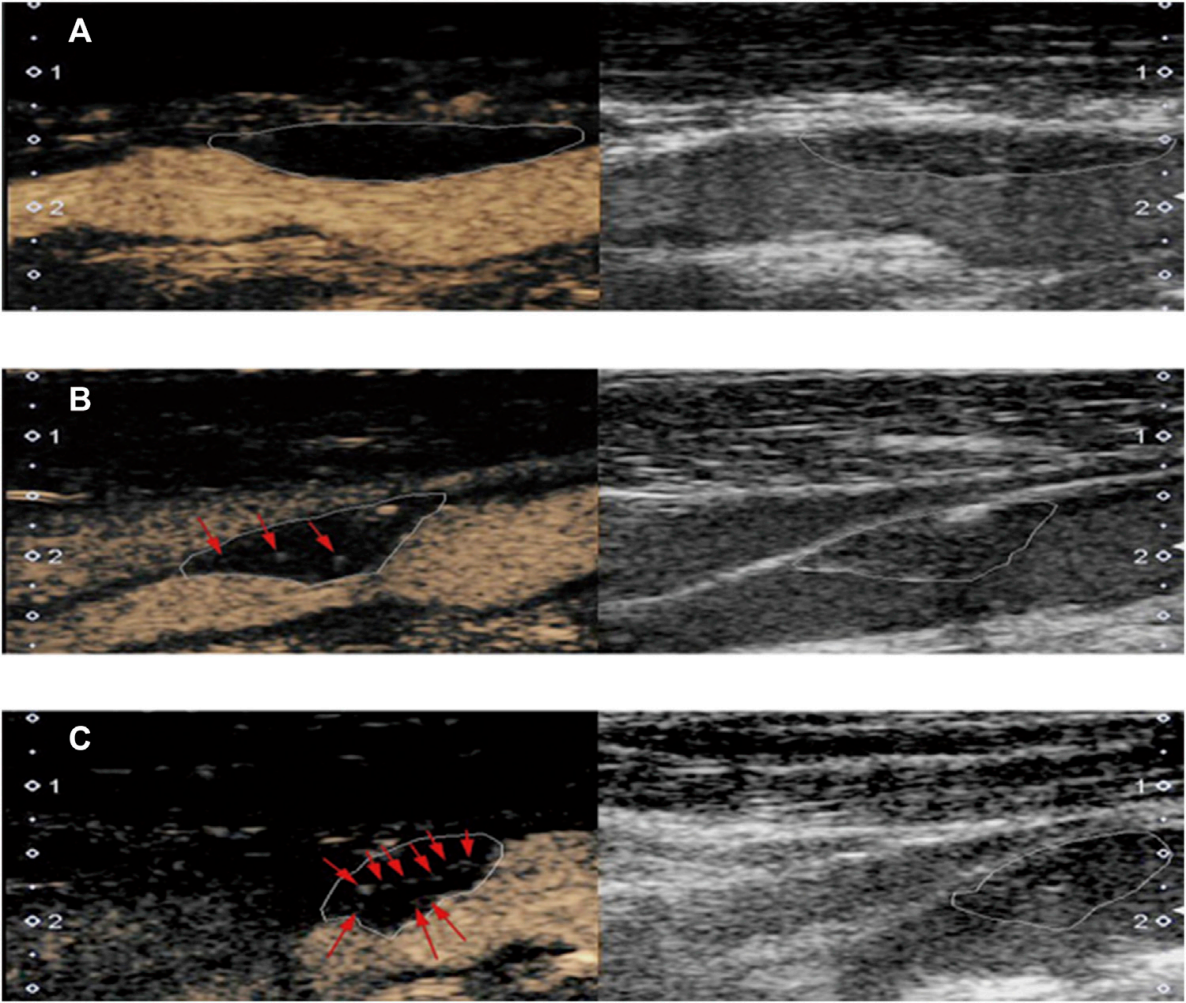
Typical examples of plaque based on contrast-enhanced ultrasonography (left) and carotid ultrasound (right). (A) grade 0: no enhancing microbubbles within the plaque; (B) grade 1: moderate enhancing microbubbles on the shoulder and/or adventitial side of the plaque; (C) grade 2: extensive enhancing microbubbles throughout the plaque.
